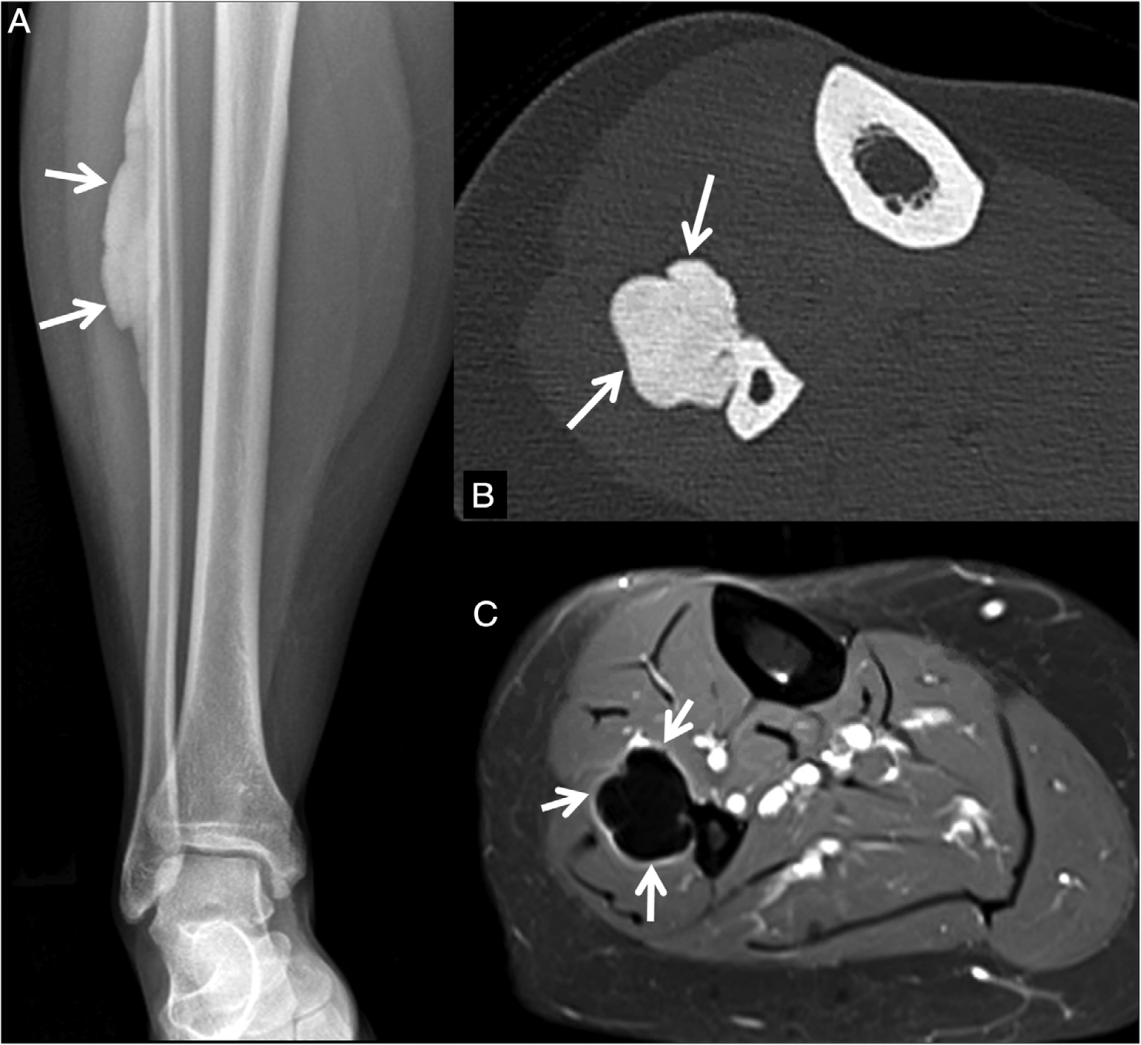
Parosteal lesion studied with radiographs, CT and MRI. A 53‐year‐old (Melo‐3) female patient with classic “dripping candle‐wax” lesion on the lateral aspect of fibular diaphysis, shown as compact bone (arrows) on plain anteroposterior X‐ray (*A*) and axial CT (*B*). Axial fat suppressed proton density‐weighted MR image (*C*) showing soft tissue edema surrounding the lesion (arrows).
